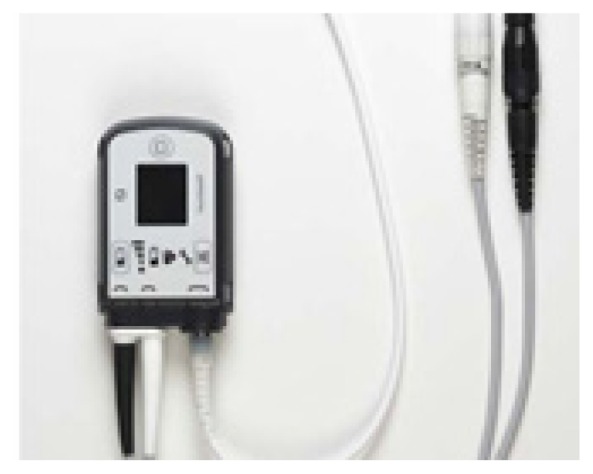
CXR*, chest radiograph; *RPM*, revolutions per minute; *HAVD*, heart assist ventricular device; *L*, liter..
